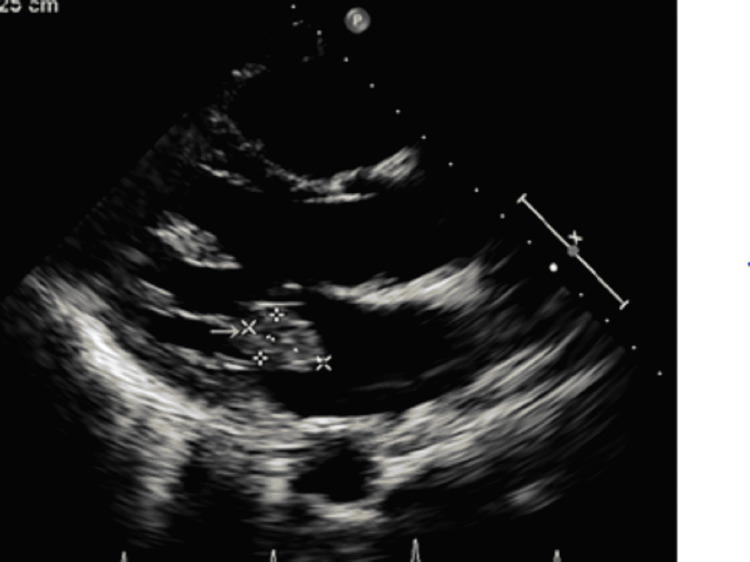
Parasternal short axis view showing mitral valve vegetation (yellow arrow)
